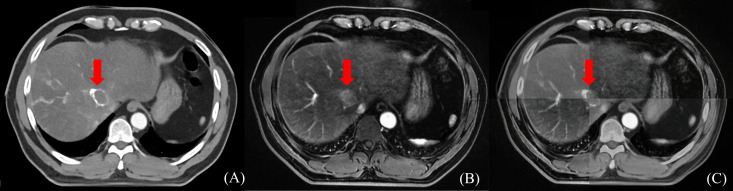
The boundary determination of HCC tumors using CT and MR registration. (A) CT contrast scan in arterial phase (B) MR T_1_-weighted arterial phase enhanced imaging (C) CT&MR rigid registration demonstration.
